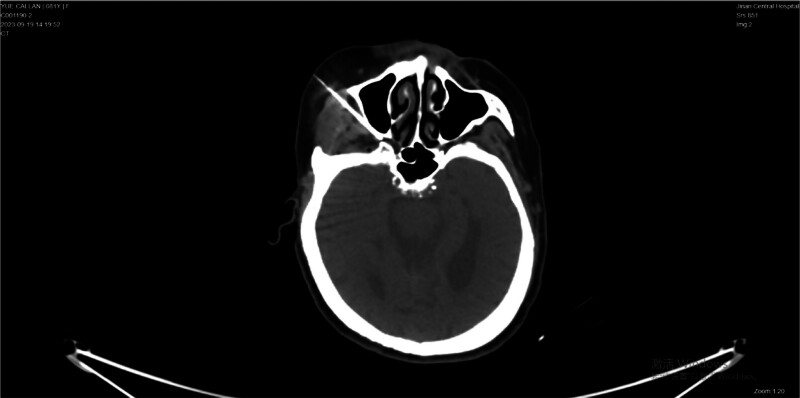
Representative CT image showing the electrode needle puncturing the pterygopalatine fossa. CT = computerized tomography.
